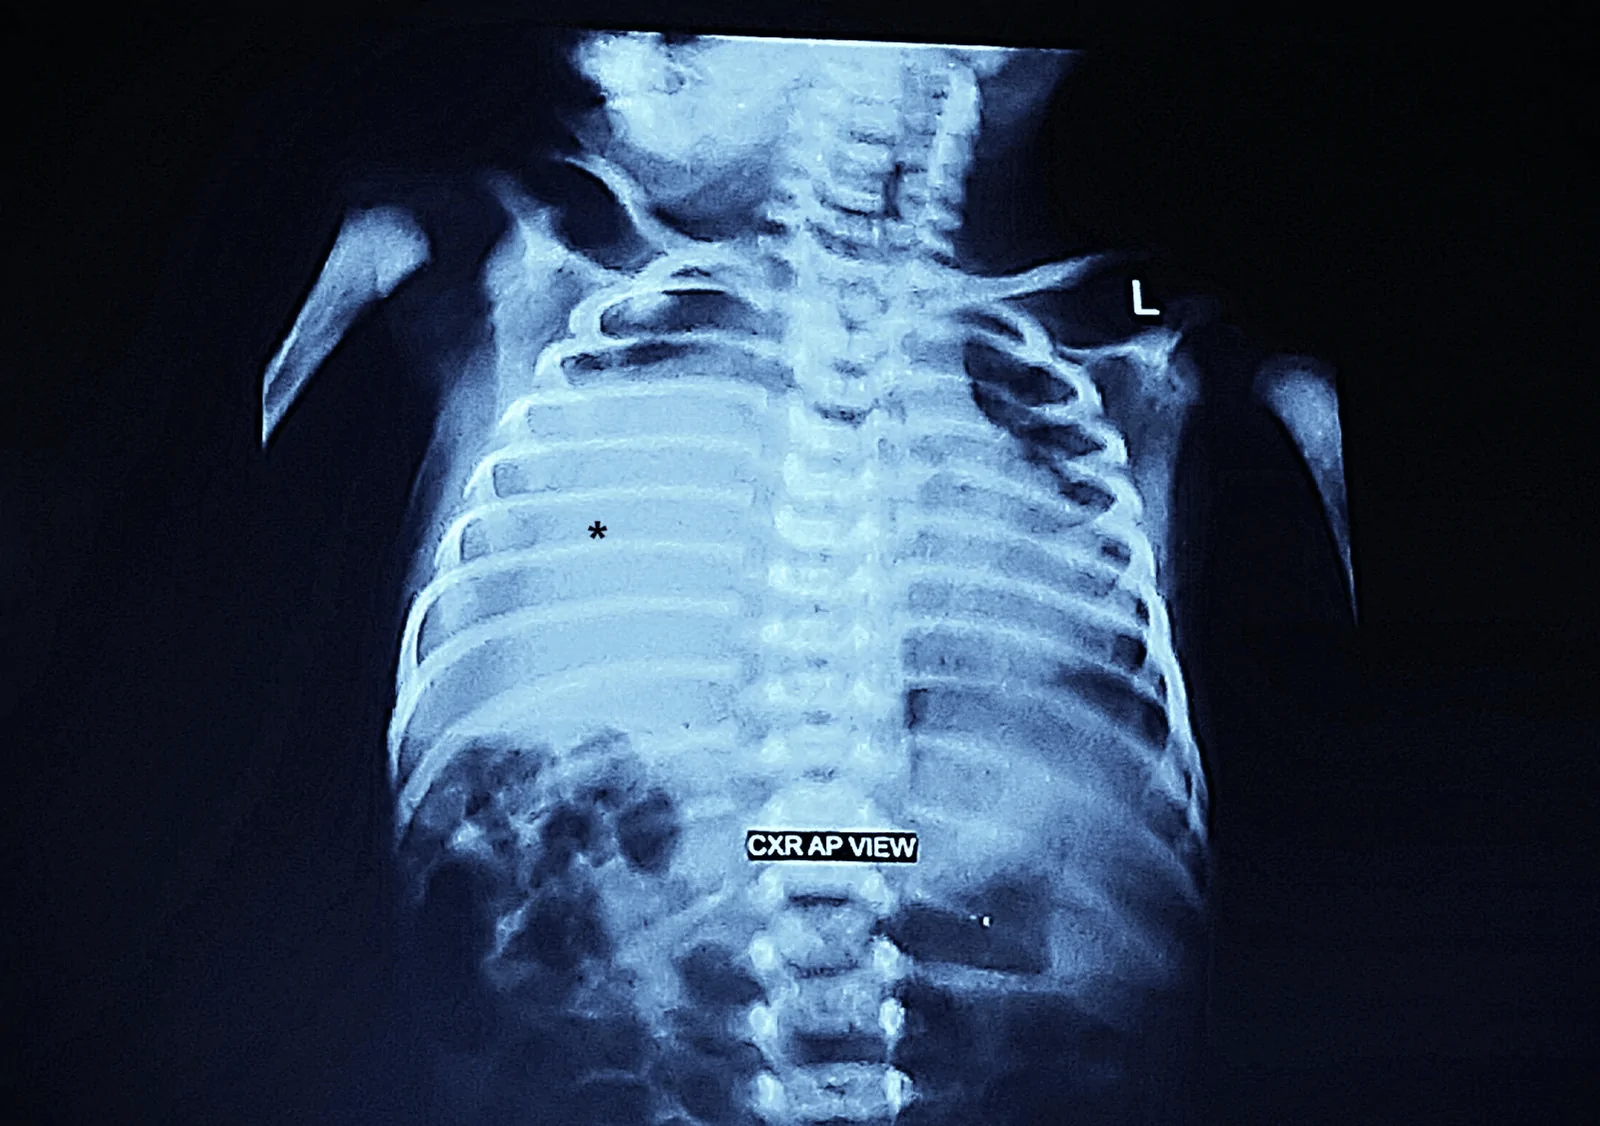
Chest X-ray (anteroposterior view) Marked elevation of the right hemidiaphragm with right lower lung compression (asterisk), consistent with right diaphragmatic eventration.
